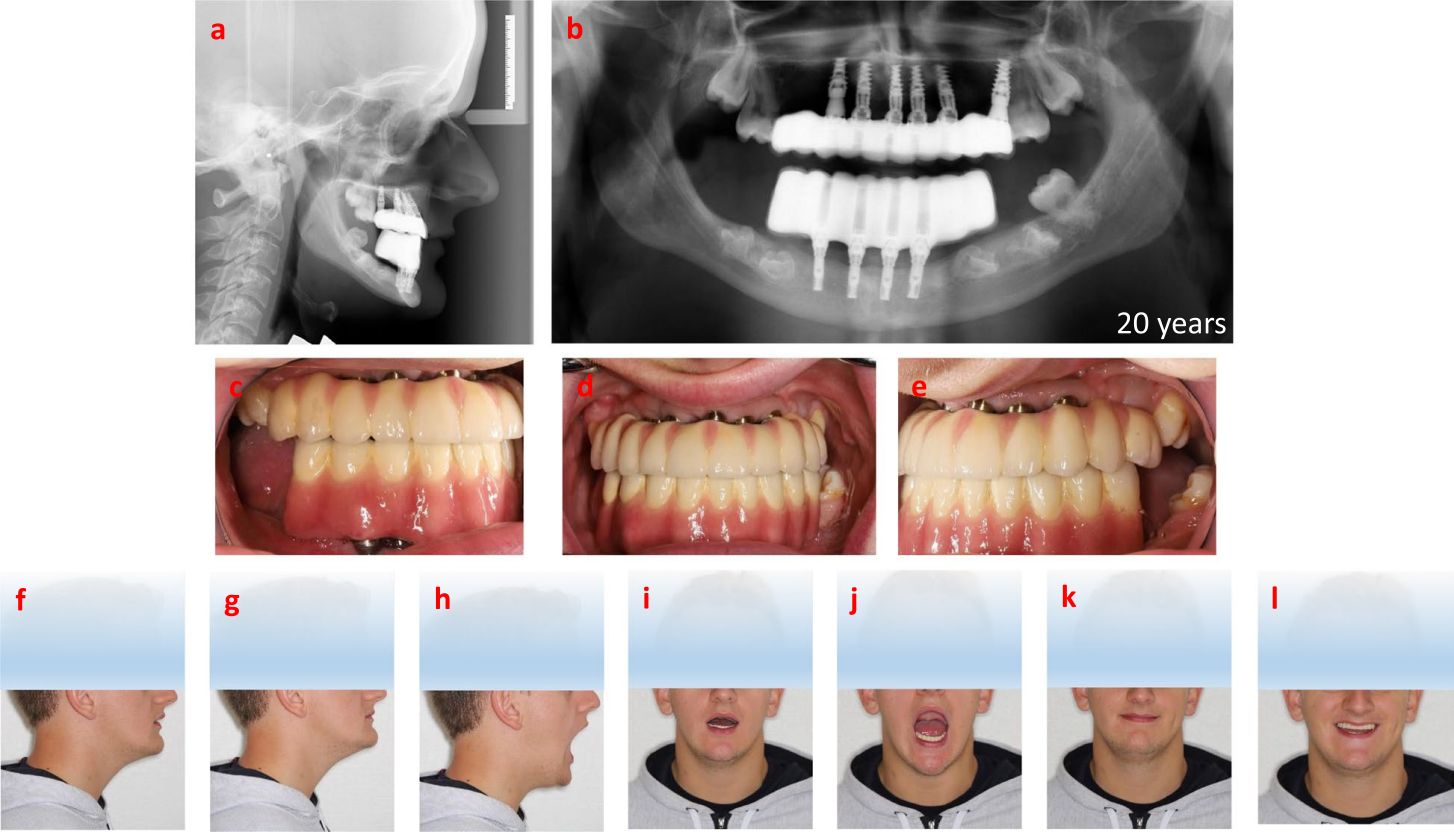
Radiographic and photographic overviews one-year post-loading of the implant-fixed bridges at the age of 20 years. a Radiographic sagittal view, and b radiographic panoramic view after one-year post-loading. c-e Photographs of dentition after one-year postloading. Sagittal and frontal facial photographs of f resting, g and k intercuspidation, h and J maximum opening, i small opening positions of lower Jaw in relation to upper jaw, and l smile line
